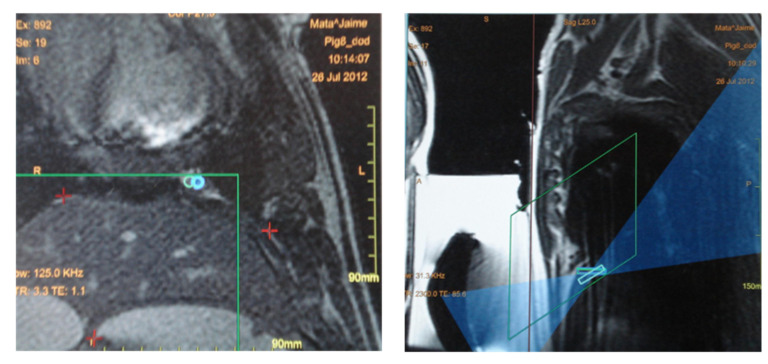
(Left) The blue circles in this coronal MRI above the diaphragm represent two focal spots planned, both localized inside the green square which represents the area available for treatment. The red crosses in the middle of the image mark the position of the diaphragm. (Right) Sagittal view of the image used for treatment planning. The rectangles represent the depth of the focal spots. The blue region corresponds to the beam path across the adjacent tissue.
